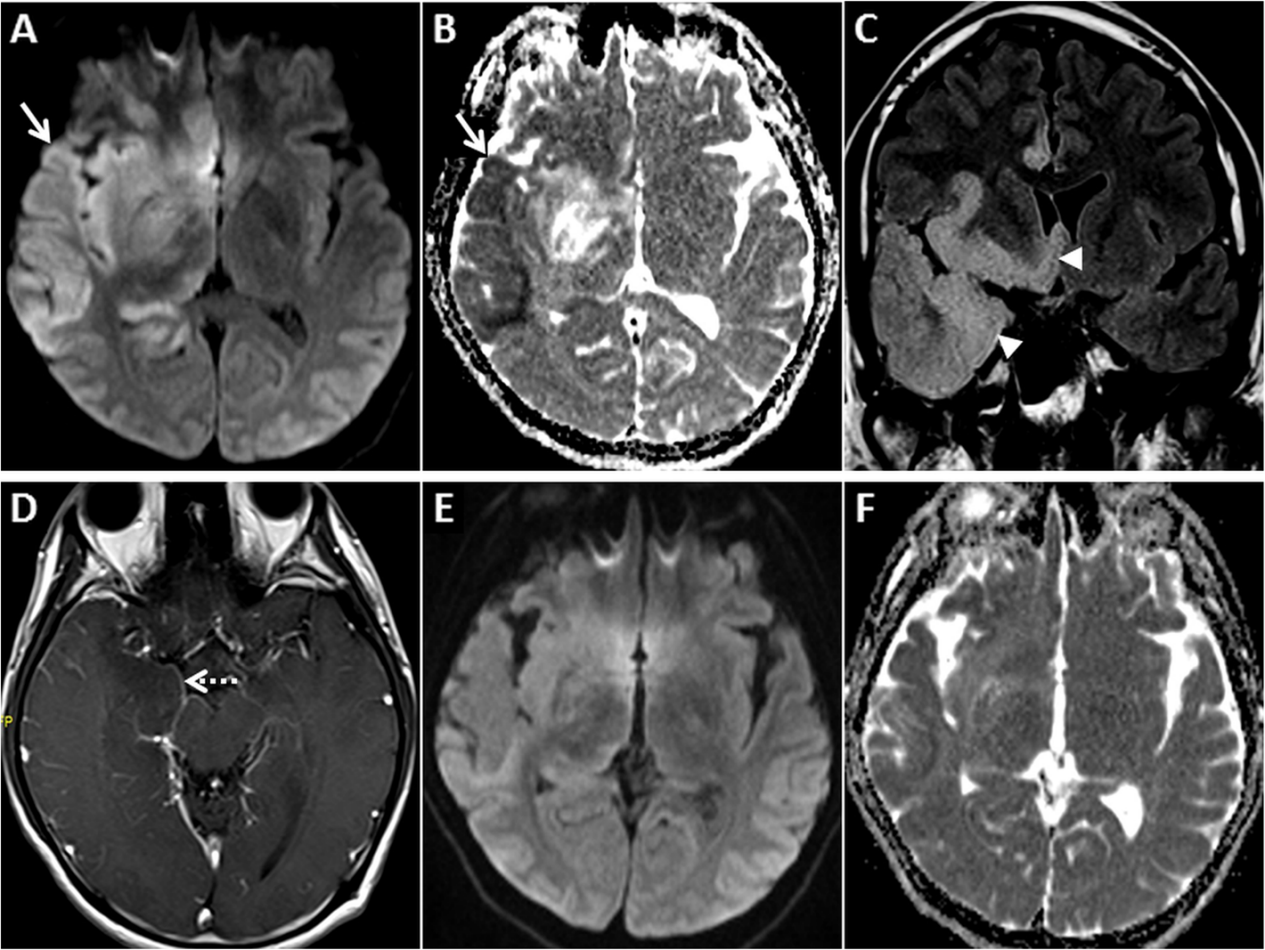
Pre- and post-contrast enhanced MRI of the brain in a 36-year-old male patient with HSVE. GRD is noted in the right temporal lobe (arrow) on axial DWI (a) and ADC image (b). Also note DWI hyperintensity in the right insula and cingulate gyrus (a, b). Coronal FLAIR image (c) reveals hyperintense signal and cortical swelling in the right inferior frontal and mesiotemporal regions (arrowheads). Post-contrast axial T1W image (d) shows smooth leptomeningeal enhancement over the right temporal cortices (dashed arrow). Post-treatment follow-up MRI shows significant resolution of previously seen GRD and cortical swelling on axial DWI (e) and ADC image (f)
